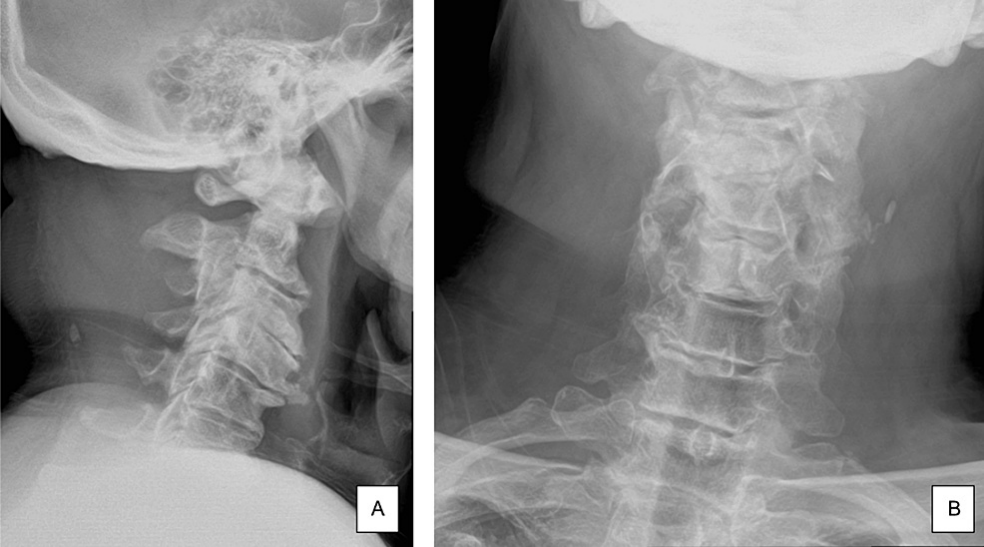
Radiographs of the cervical spine demonstrating severe degenerative changes at C3-C4. (A) Sagittal. (B) Anteroposterior.
